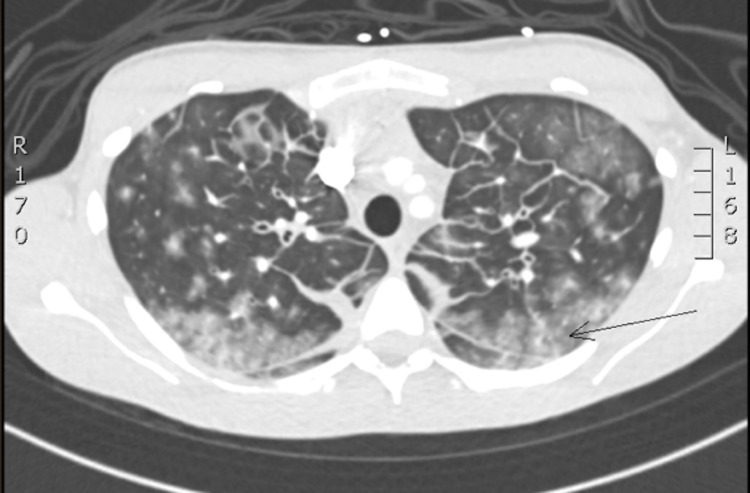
CT Chest Pulmonary Angiogram demonstrating non-cardiogenic pulmonary edema. (black arrow)
